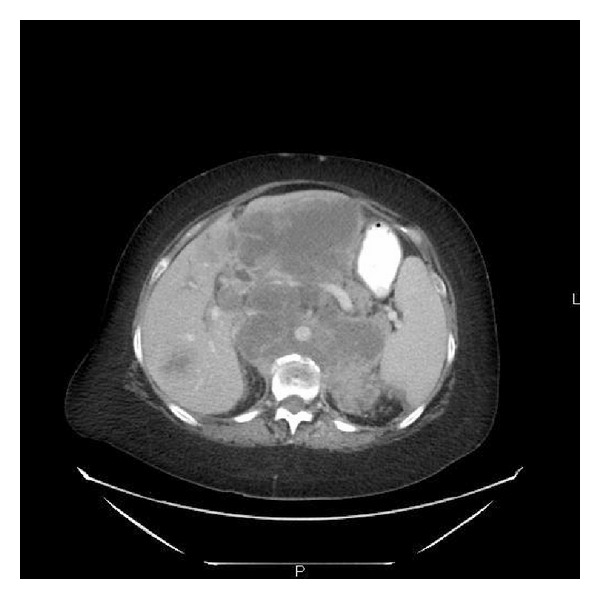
Computed tomography of abdomen showing a big retroperitoneal mass engulfing the vascular structures in the abdomen.
